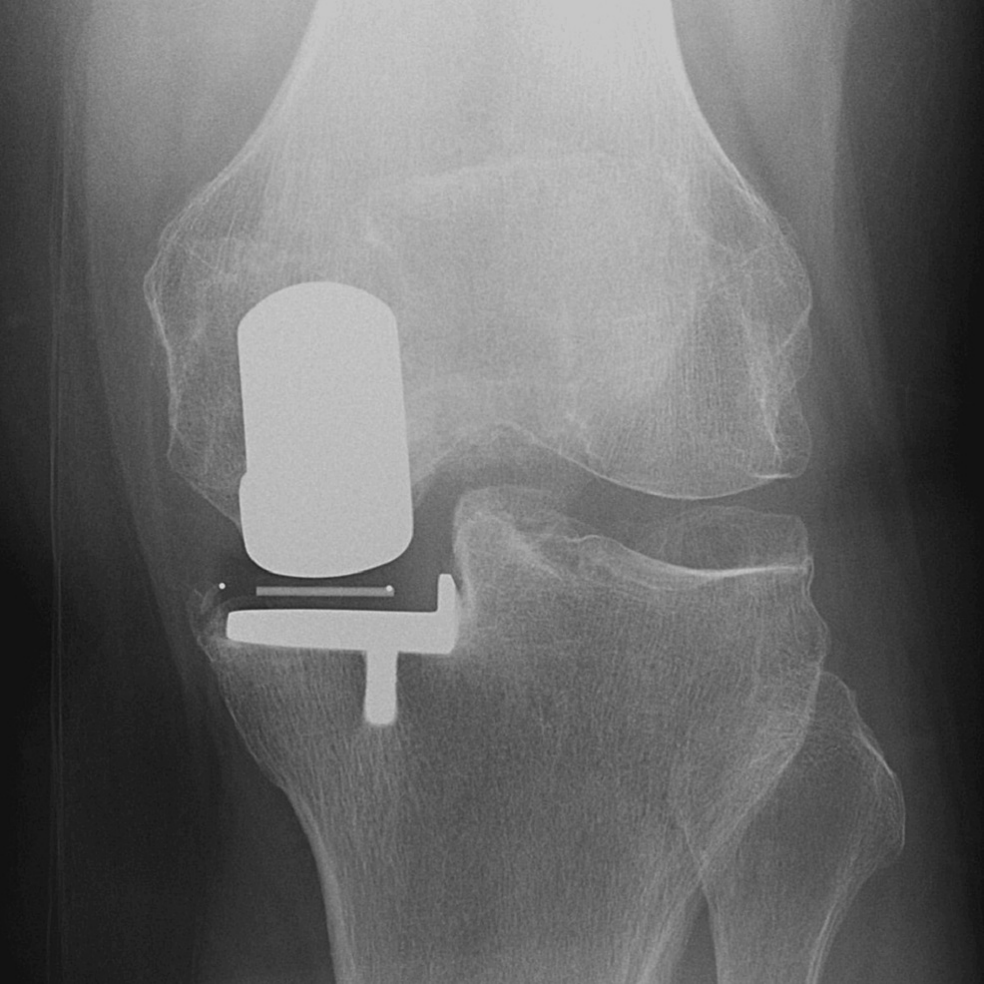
Radiography at two years postoperatively.
